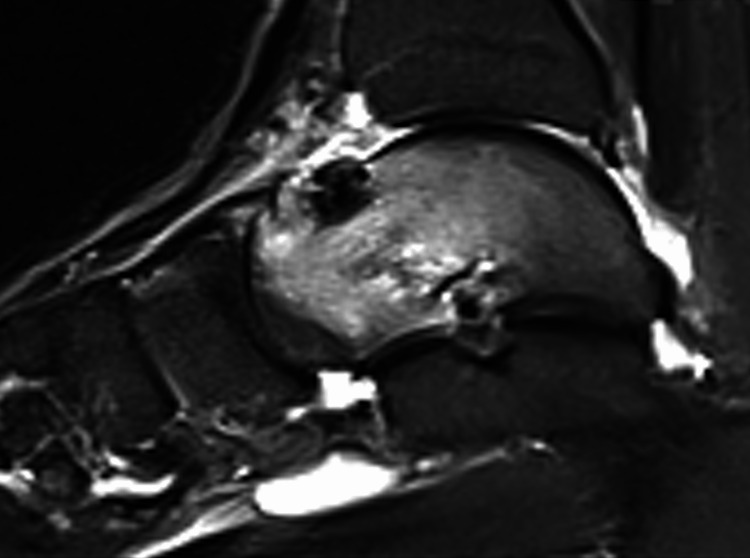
The patient's review with an MRI scan a year after the onset of his symptoms. Apart from the osteoid osteoma-related nidus, a fracture line spanning from the anterosuperior to the posteroinferior part of the talar neck is also evident.
